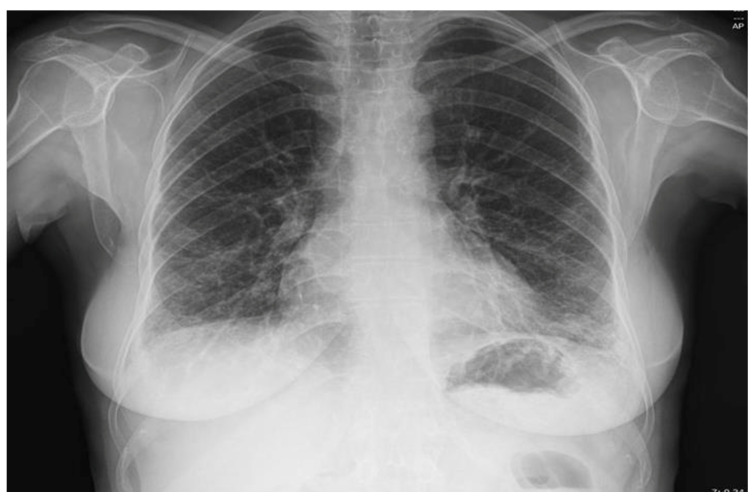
Chest X-ray showing a more accentuated bilaterally basal reticular lung pattern.
